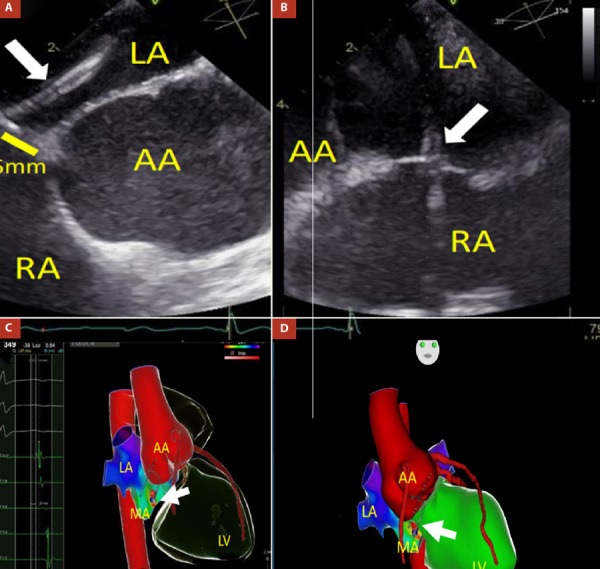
(A and B) Transseptal puncture guided by transesophageal echocardiography: transseptal sheath and needle (white arrow) are directed from RA to LA and located 5 mm from AA. Three-dimensional Electroanatomic Mapping fusioned with computed tomography shows a catheter ablation positioned in the poster-septal region of the mitral annulus. C) Right anterior oblique (RAO) view: Local activation time (LAT) was -30ms from the atrial coronary sinus electrogram, as determined from both bipolar and unipolar electrograms. D) Anteroposterior (AP) view: Radiofrequency catheter ablation (white arrow) in the posteroseptal region of the mitral annulus, near to dilated aortic root.
